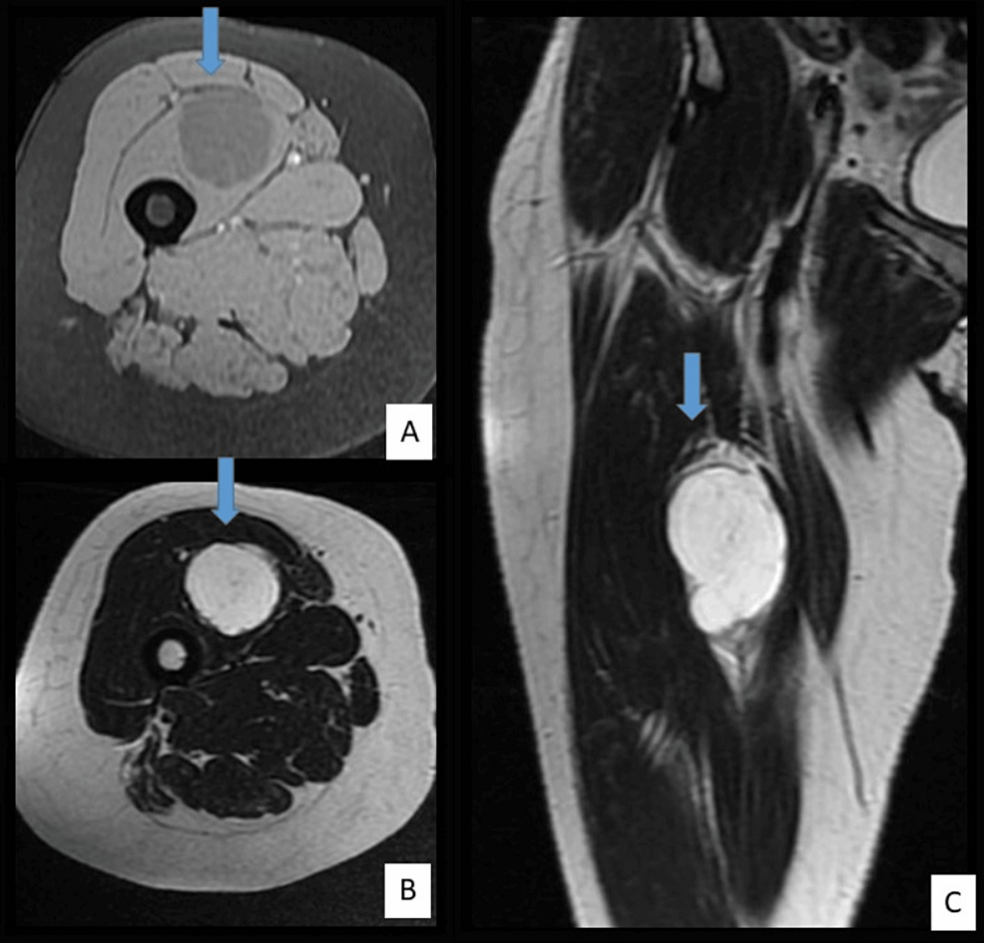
MRI images of the right thigh; A: Axial T1-weighted image showing the well-defined tumor in hypointensity. B: Axial T2-weighted image showing the well-defined tumor in hyperintensity. C: Frontal T2-weighted image showing the tumor in hyperintensity and well-defined.
